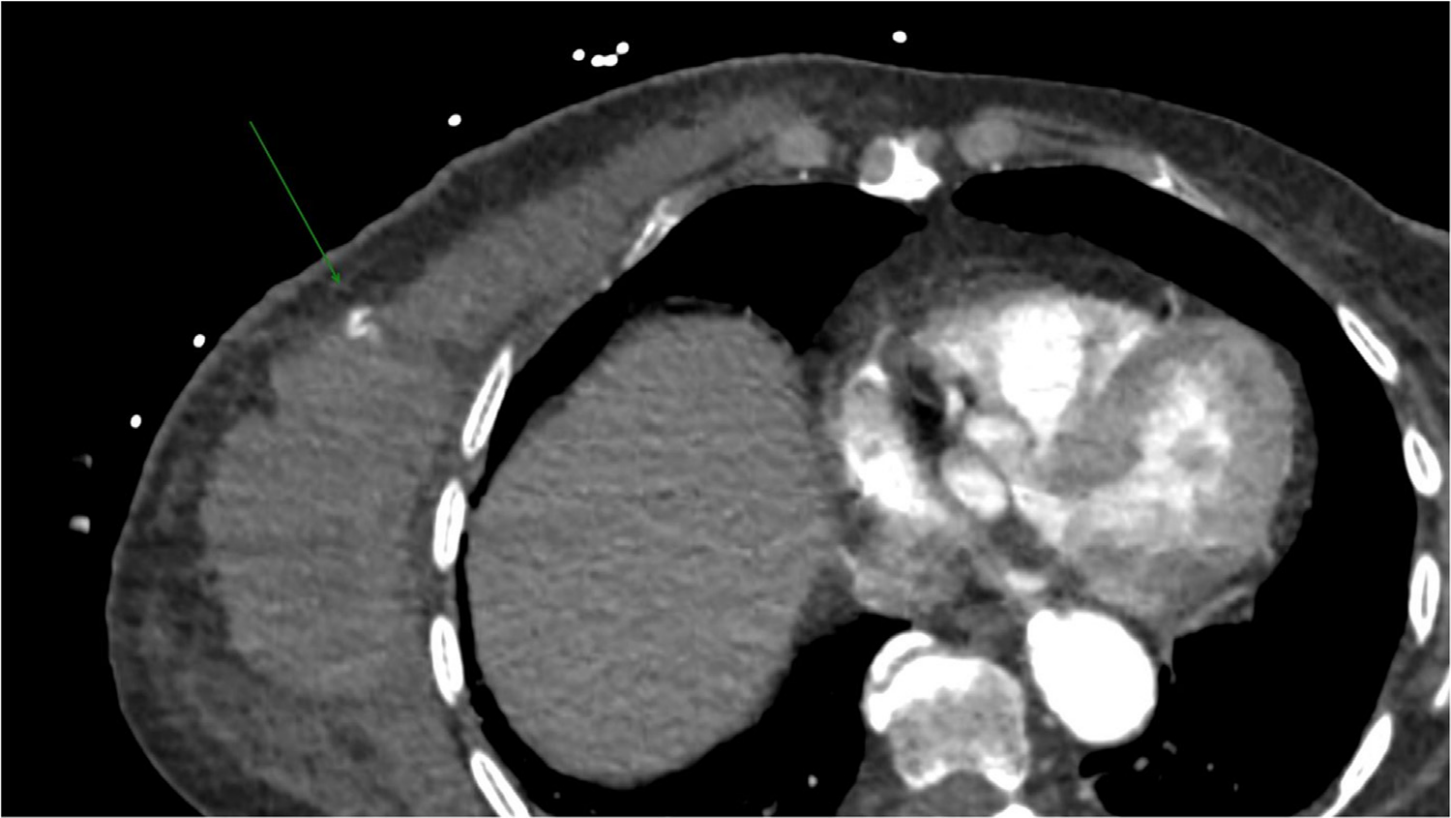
The computed tomography revealed an active bleeding from the superficial skin flap.
